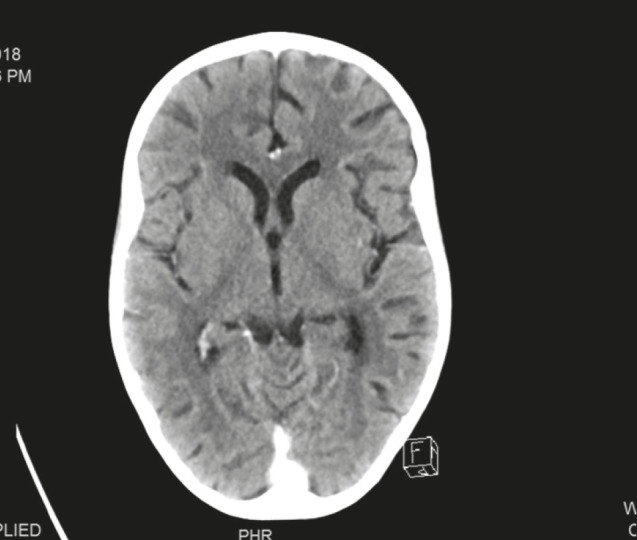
Computed tomography brain scan showing involution with brain
